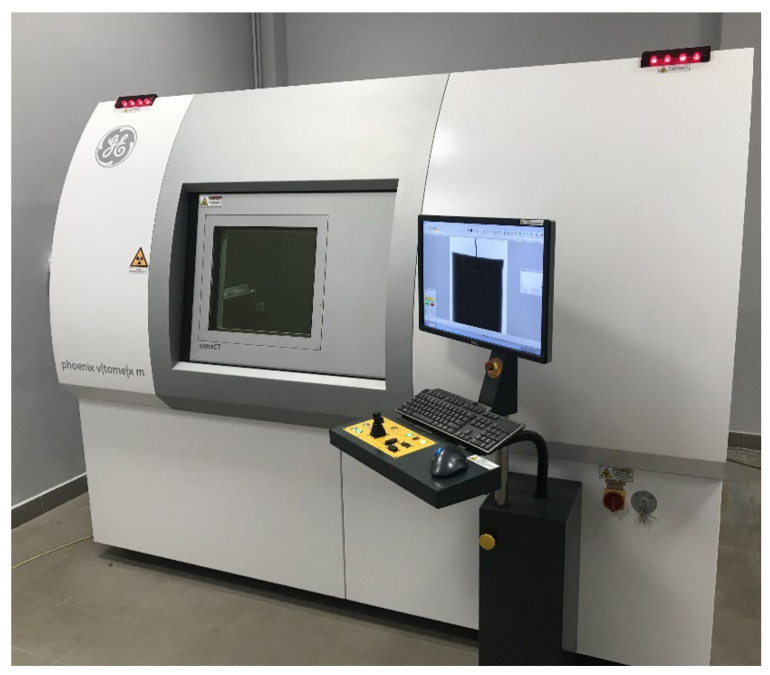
X-ray CT scanner GE phoenix v|tomex|m.
